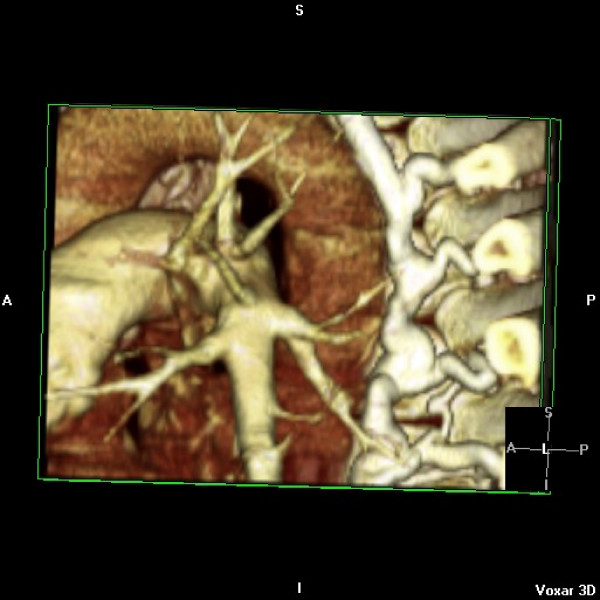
Contrast CT angiogram of left pulmonary artery showing a "wispy" ill defined embolus in the upper lobar artery.
